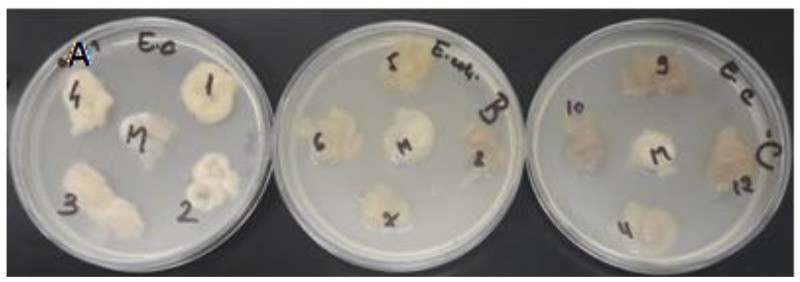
Antimicrobial activity of wool samples against *Escherichia coli* ATCC 25922: A—wool samples treated with citric acid; B—UV-irradiated wool samples; C—untreated wool samples; 1—wool dyed with aqueous extract, 2—wool dyed with ultrasonic extract; 3—wool dyed with alcohol extract; 4—wool dyed with acid extract, M—control.
